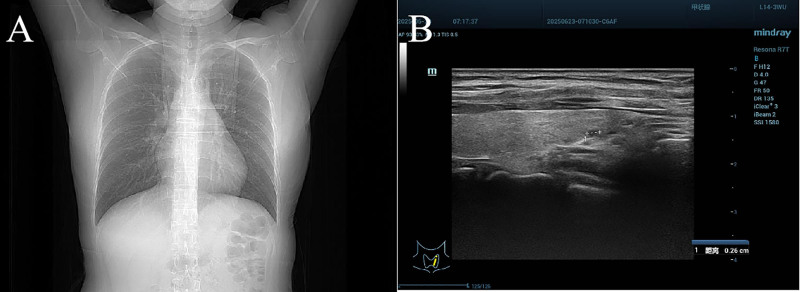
Representative imaging of chest CT and thyroid ultrasound. (A) Representative imaging for pulmonary micronodules by chest CT; (B) representative imaging for thyroid cystic nodule via thyroid ultrasound. CT = computed tomography.
